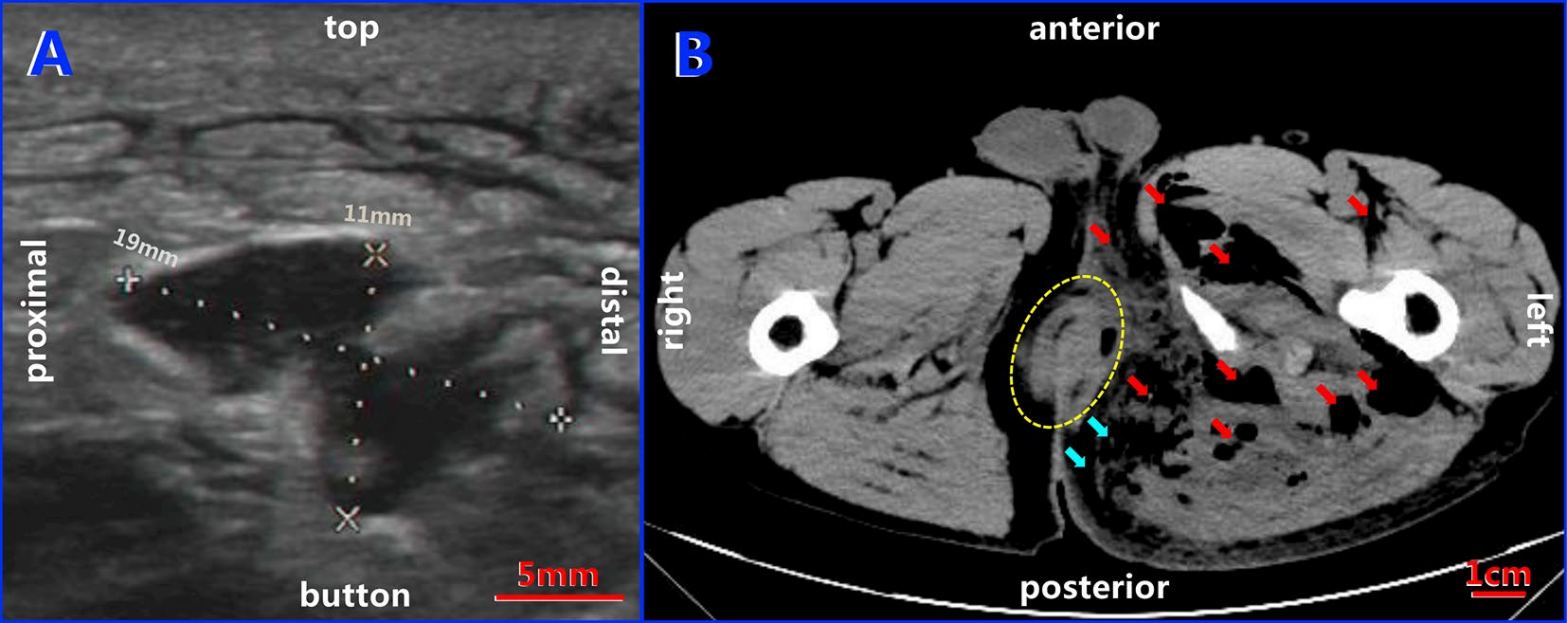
Ultrasound and CT images of the buttocks showing gas-producing perianal infection. A Anechoic area (1.9 cm × 1.1 cm) detected by ultrasound with a thickened subcutaneous layer. B Multiple liquid and gas zones (red arrows) and gas–liquid planes detected in the muscle spaces in the left hip on the transaxial CT plane
